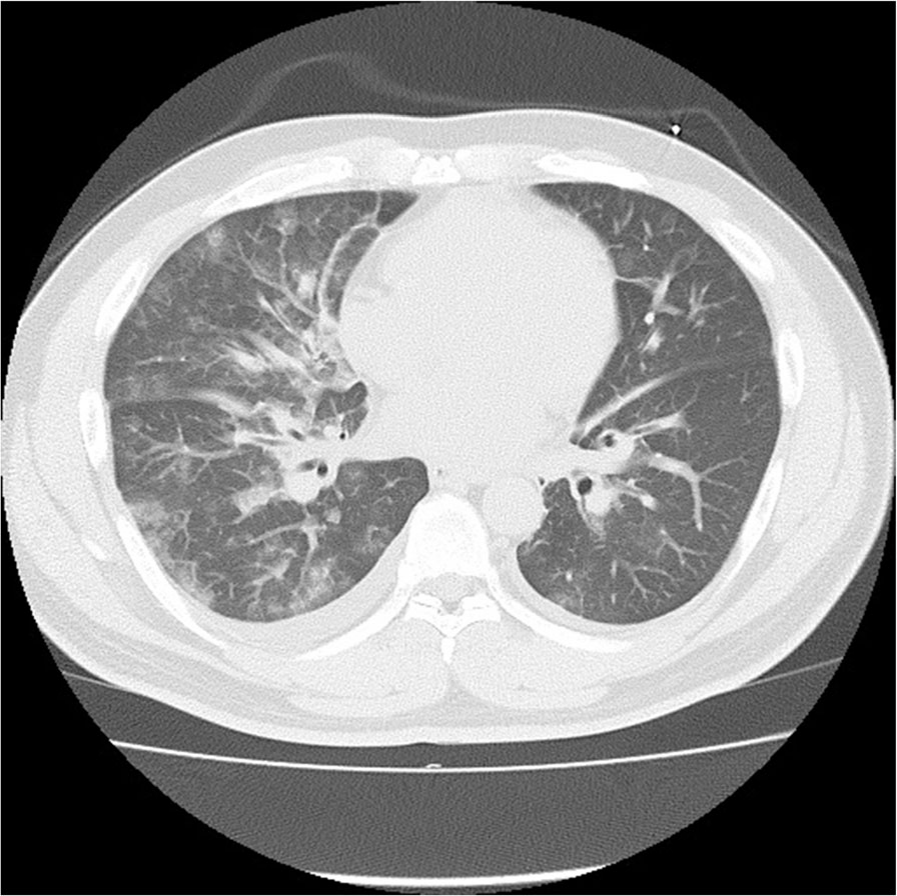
Chest computed tomography showed ground glass opacity with diffuse lobular and septal wall thickening
